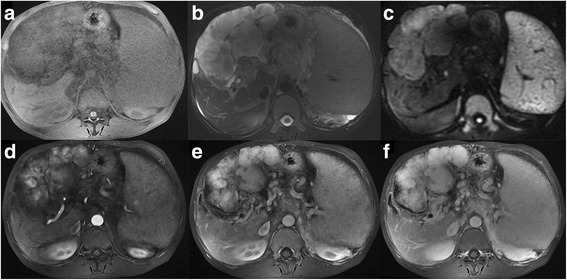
Abdominal conventional and contrast-enhanced MRI scans displayed a soft tissue mass and T1WI was isointense, compared to the muscle signal (a). Transverse T2WI showed slight hyperintensity (b). The DWI sequence showed slightly hyperintensity (c). Transverse artery phase showed the central region enhanced (d). In the venous phase (e) and delayed phase (f), the enhanced range increased, showing centrifugal enhancement
